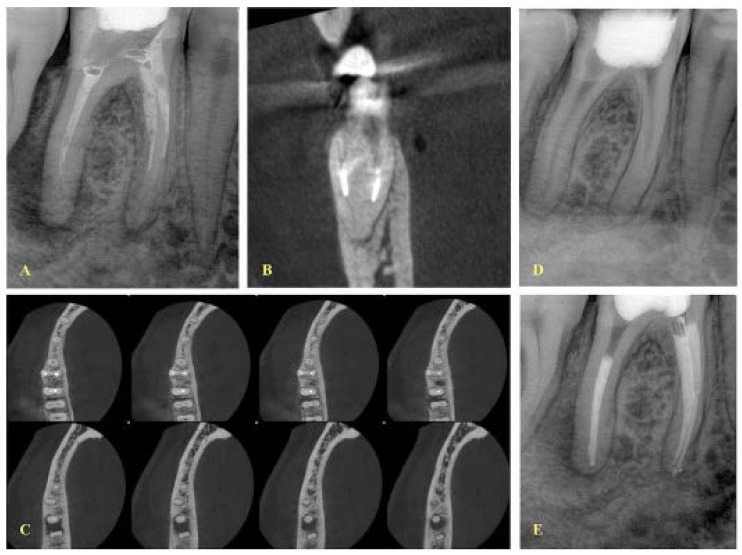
(A) Initial periapical radiograph (mesial angle view) of a mandibular first molar with periapical lesions and an instrument fracture at the middle third of the mesial root. The fragment is located at the mesiobuccal canal, according to the buccal-object rule. (B) CBCT coronal view indicating that the two canals are separate, (C) axial views demonstrating that the two canals are separate, however the two apical foramina are very close to each other, (D) radiograph after the removal of the fragment. (E) Final radiograph.
